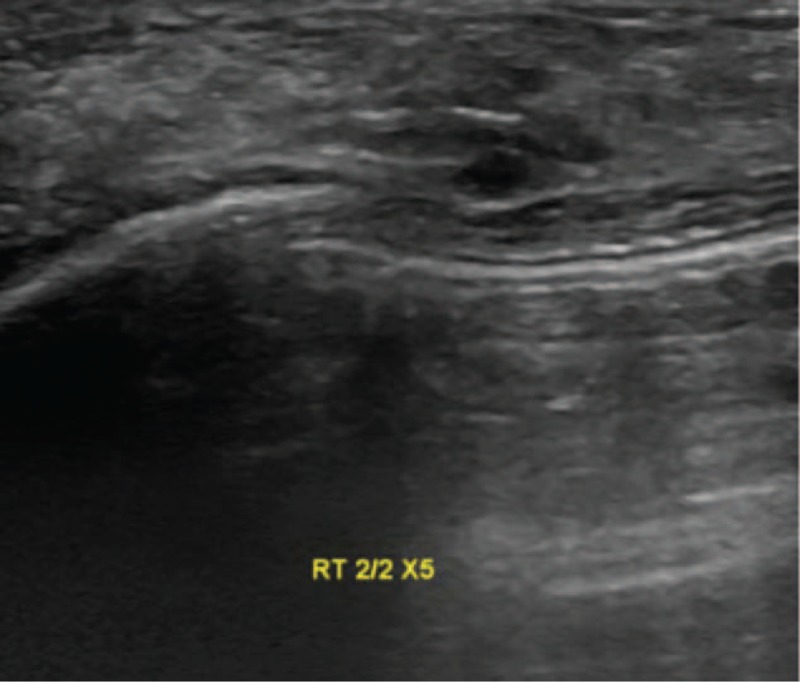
Ultrasound examination and percutaneous ultrasound-guided biopsy of the right breast lump.
